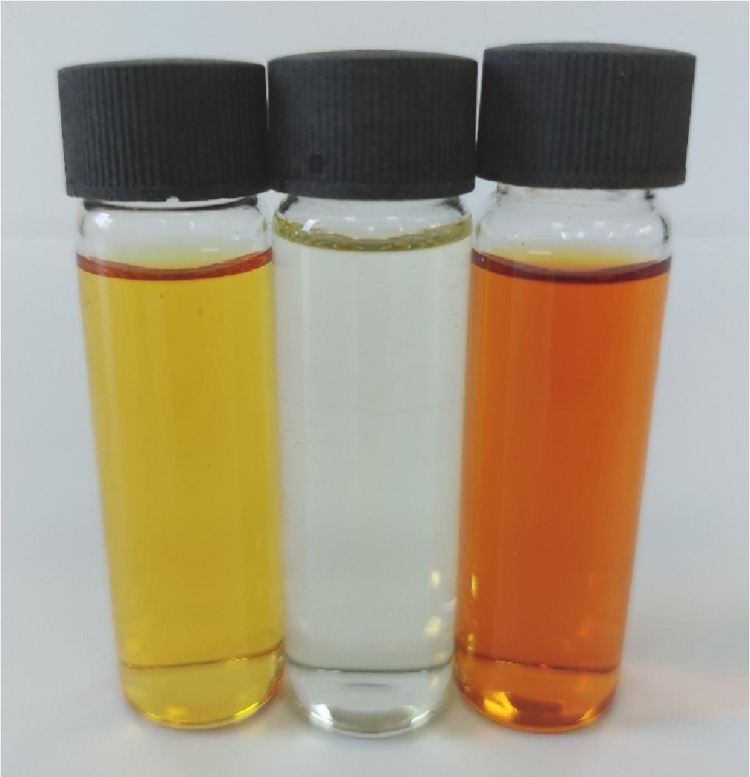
Color change upon mixing CLA acceptor (*far left; brown*) with the donor (*middle; colorless*) to generate the CT complex (*far right; intense brown*). (For interpretation of the references to color in this figure legend, the reader is referred to the web version of this article.)
